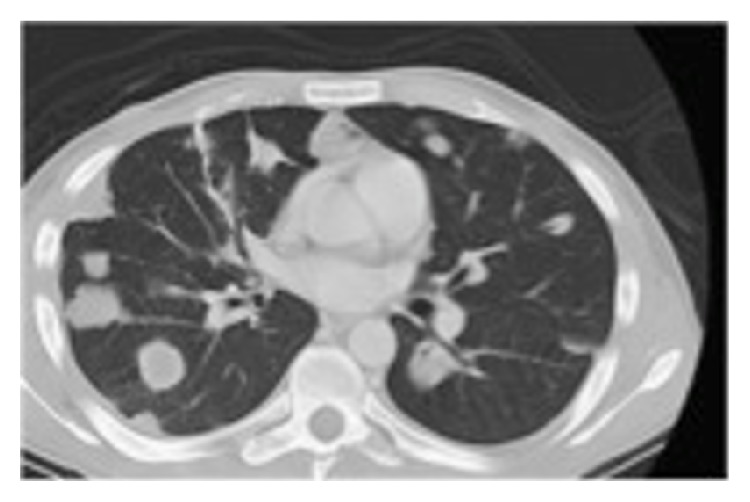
CT Chest. CT chest with diffuse pulmonary nodules.
